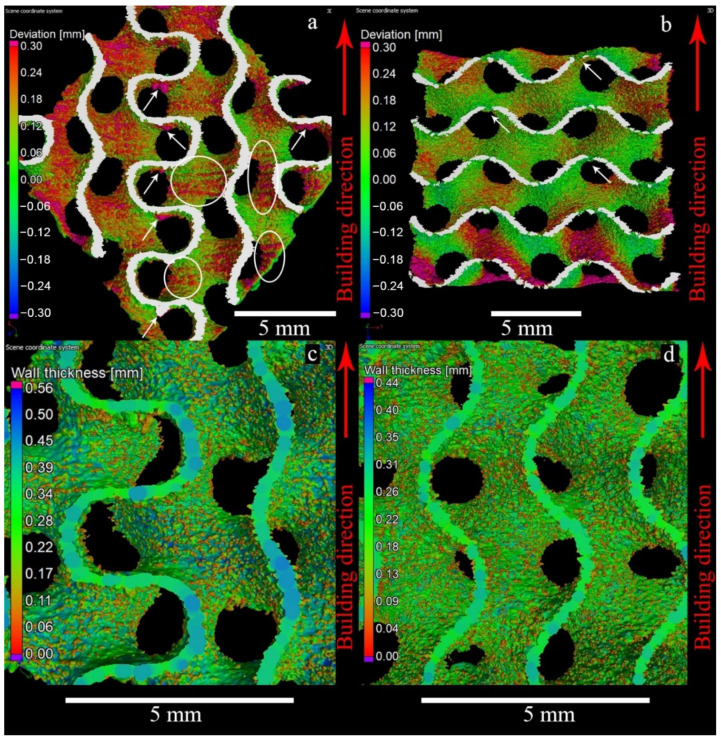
3D rendering of the CT reconstructions for the Nominal/Actual Comparison of the MT gyroid (a) and WT gyroid (b). Visualization of the wall thickness for the MT gyroid (c) and WT gyroid (d). White arrows indicate stalactite-like structures on the horizontally oriented parts of the MT walls (areas parallel to the layers, purple color). The white circles highlight the partially melted powder particles attached to the surface of the vertical areas.
